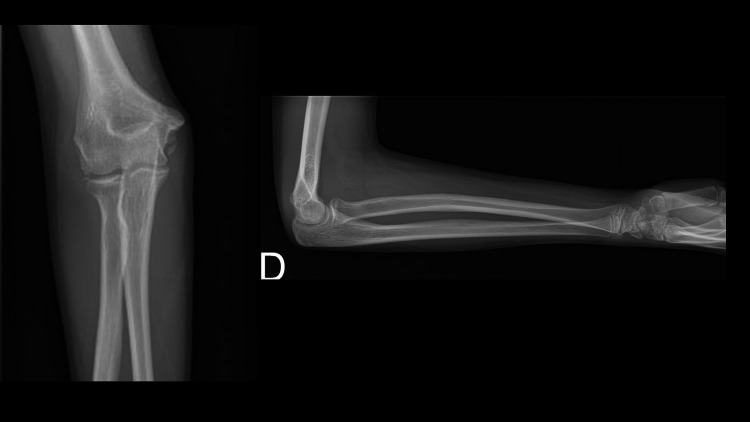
Radiographs of the elbow at the one-year follow-up.
